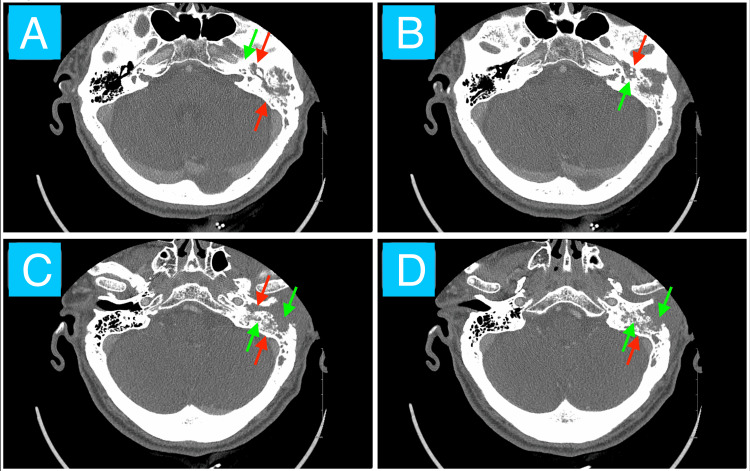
Non-contrast CT of the head Axial CT of the head without contrast from most superior (image A) to most inferior (image D) in a 63-year-old male showing cavitary opacification (left middle ear, EAC, mastoid bone) (red arrows) and destructive bony changes (coalescent mastoiditis, erosion of mastoid and tympanic segments of facial nerve canal) (green arrows). These findings are suspicious for an aggressive mass. Biopsy confirmed moderately differentiated SCC. EAC: external auditory canal; SCC: squamous cell carcinoma
